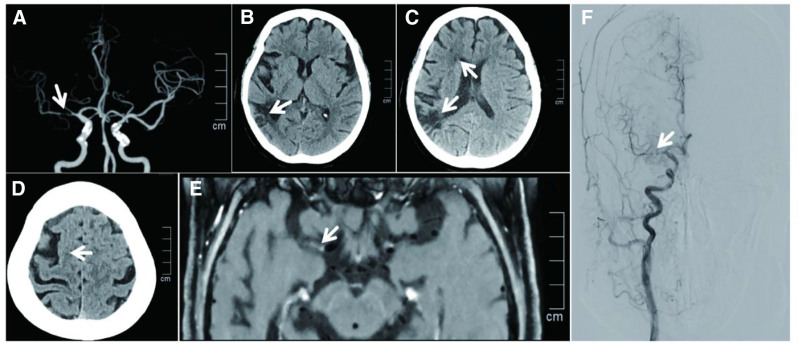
Preoperative CT, DSA, and high-resolution MRI findings. (A) CTA reveals severe stenosis in the right middle cerebral artery; (B) Right posterior watershed cerebral infarction; (C) Right anterior and posterior watershed infarction; (D) Right cerebral atrophy; (E) High-resolution MRI: shows diffuse severe stenosis of the right M1 segment with wall enhancement, indicative of an unstable plaque; (F) Preoperative DSA demonstrates severe stenosis in the M1 segment of the right middle cerebral artery (video0000BF05). CT = computed tomography, CTA = cranial computed tomography, DSA = digital subtraction angiography, MRI = magnetic resonance imaging.
